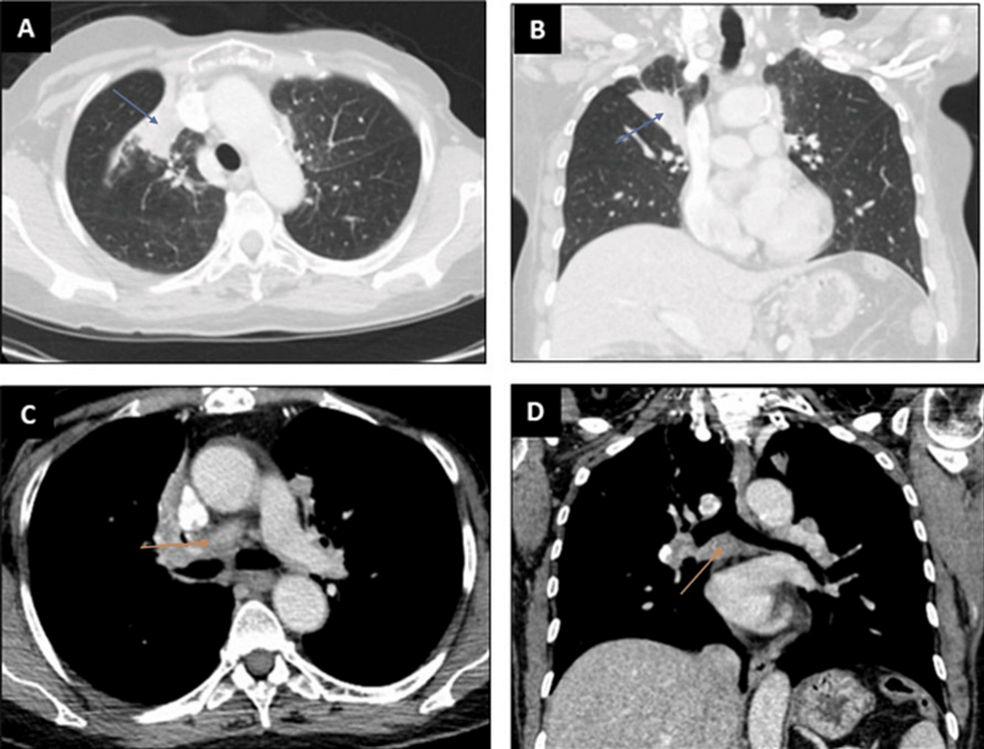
CT of the chest showing lung lesions and lymphadenopathy. (A, B) Axial and coronal view on CT scans (parenchymal window) showing right upper lobe lesion. (C, D) Axial and coronal view on CT scans showing mediastinal and hilar lymphadenopathy. (A, B) Blue arrow showing a lesion in the right upper lobe. (C, D) Orange arrow showing mediastinal lymphadenopathy.
